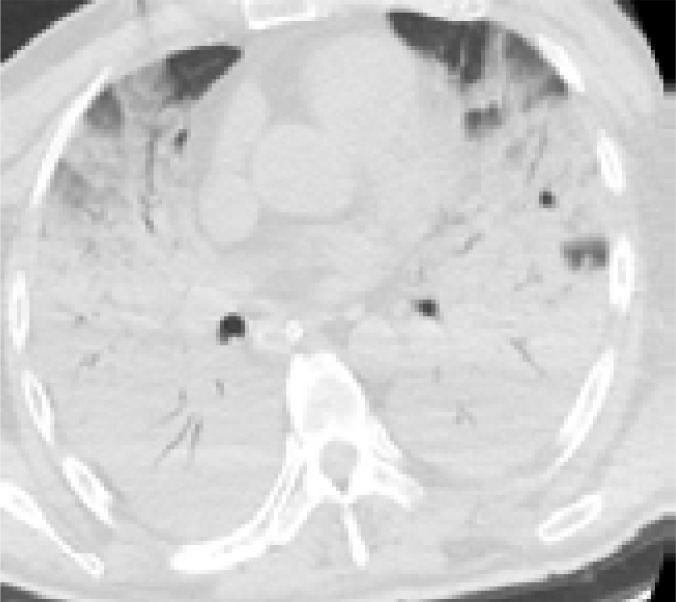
G) A 77-year old male patient with DM+HT history applied to hospital with complaints of cough, fever, and shortness of breath for 10 days. Dyspnea increased more specifically in the last 24 hours. Density increase in ground glass density, more specifically in the lower lobes, in both lungs, and interstitial and interseptal thickening, more specifically in partly peripheral segments, were found in chest CT image of the patient. (reticular ground glass areas /crazy paving pattern) (They are indicated in Black Oval shapes)
